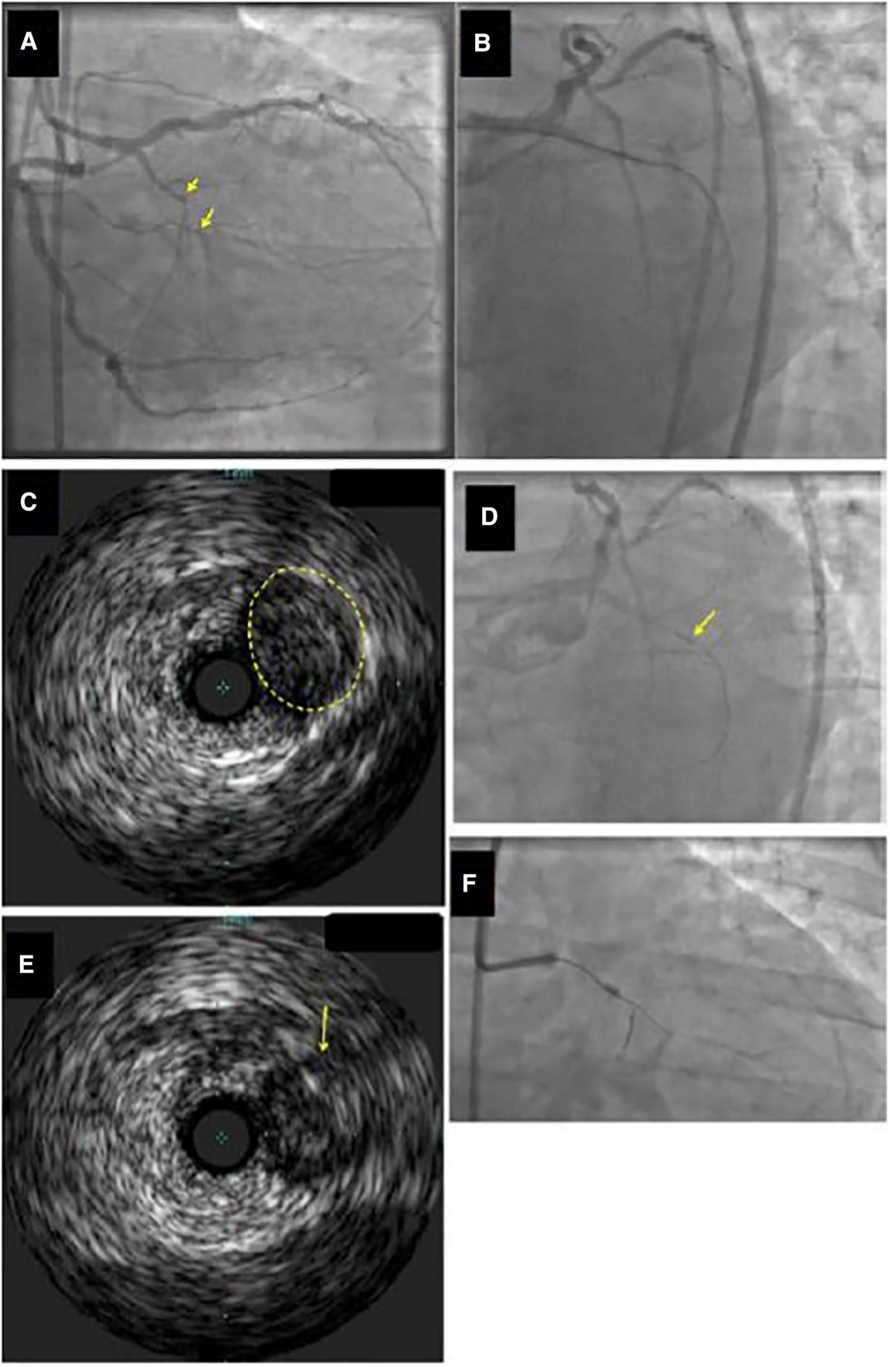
Example of identification of the proximal cap location by intravascular ultrasound (IVUS). (Panel A) Ostial chronic total occlusion (CTO) of the first obtuse marginal branch (arrow). (Panel B) The guidewire kept entering the distal circumflex during antegrade wire escalation approach. (Panel C,D) IVUS demonstrated that the CTO (yellow circle in Panel C) originated proximal (arrow in Panel D) to the distal circumflex's apparent origin. (Panel E) A Confianza Pro 12 guidewire was used for antegrade crossing and its location within the CTO was confirmed by IVUS. (Panel F) Confianza Pro 12 was advanced through the occlusion. Modified with permission from Brilakis ES. Manual of chronic total occlusion interventions a step-by-step approach. Second edition. ed. London: Elsevier/Academic Press; 2018.
